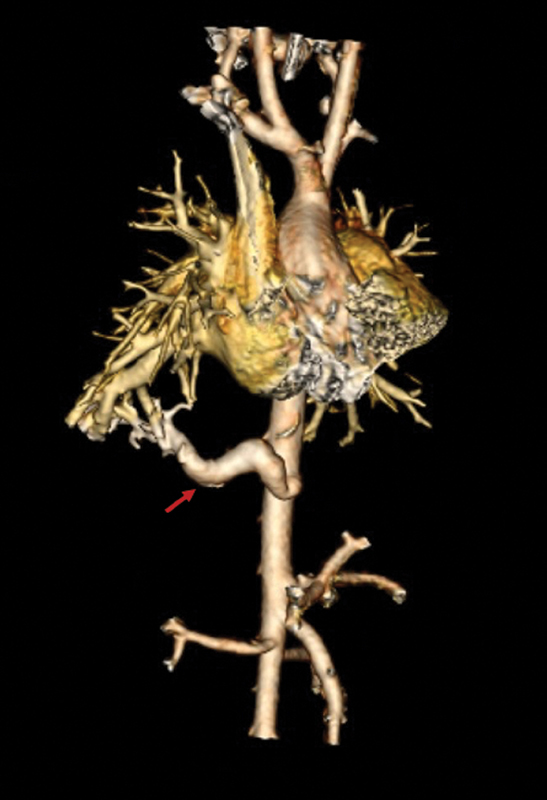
Computed tomography reconstruction demonstrating the vascular anatomy of the pulmonary sequestration. Feeding artery noted by red arrow.
